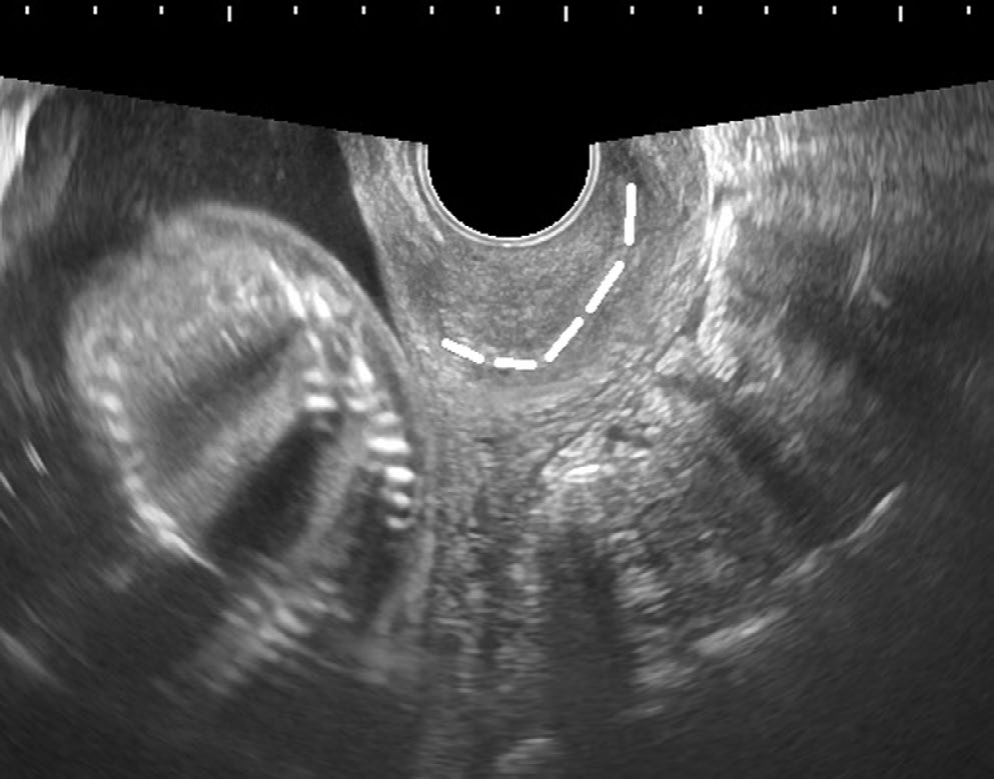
Transvaginal ultrasonography at 20 wk of gestation (after manual reduction). The cervix (dotted white line) is positioned normally and is not stretched, contrary to its previous appearance
